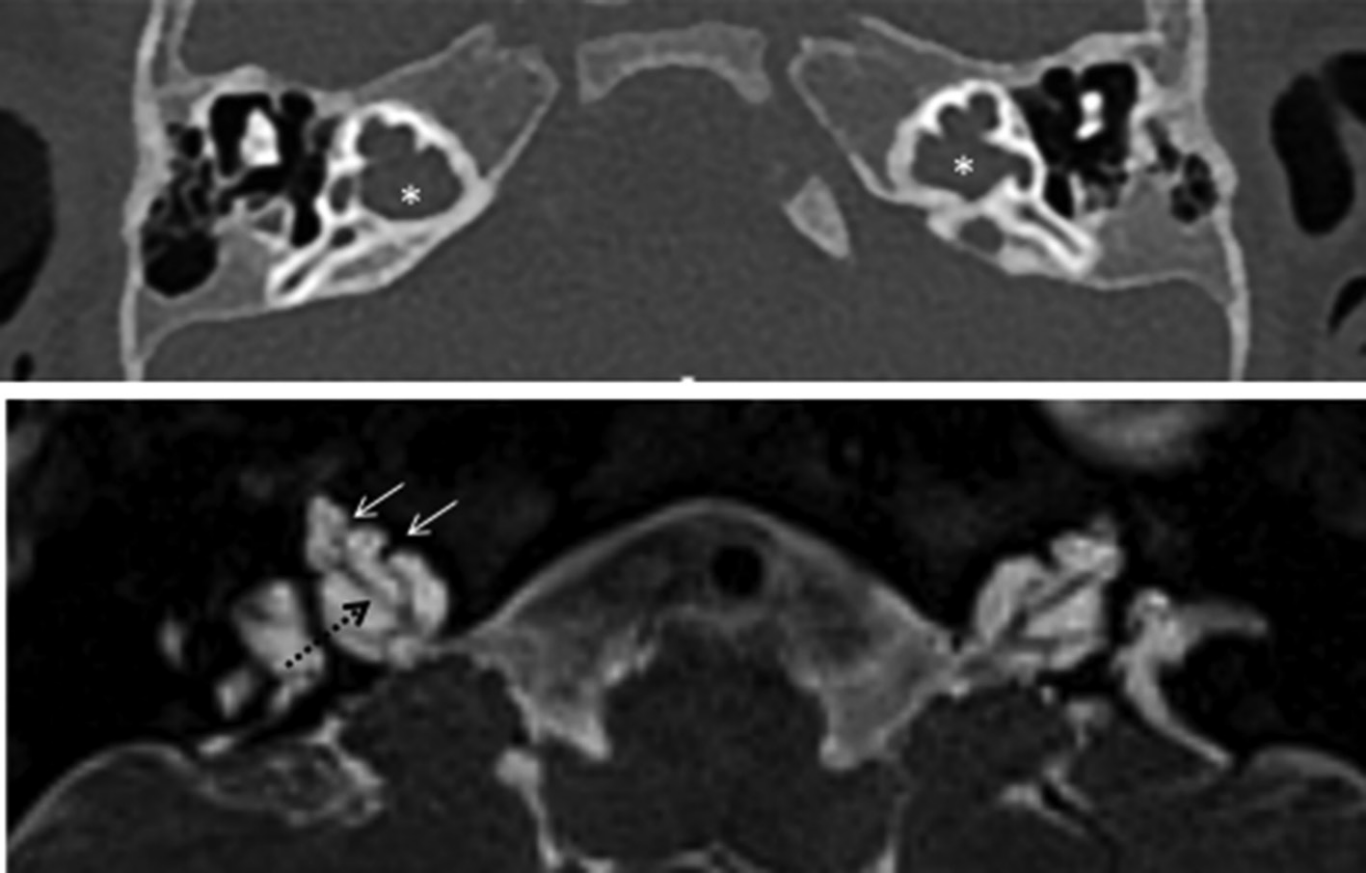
Typical CT (up) and MRI (down) appearances of incomplete partition Type 3. Note the enlarged internal auditory meatus (*), the presence of cochlear nerves (dotted arrow) and the preserved interscalar septi accounting for the typical shape of the cochlea in these patients (arrows).
